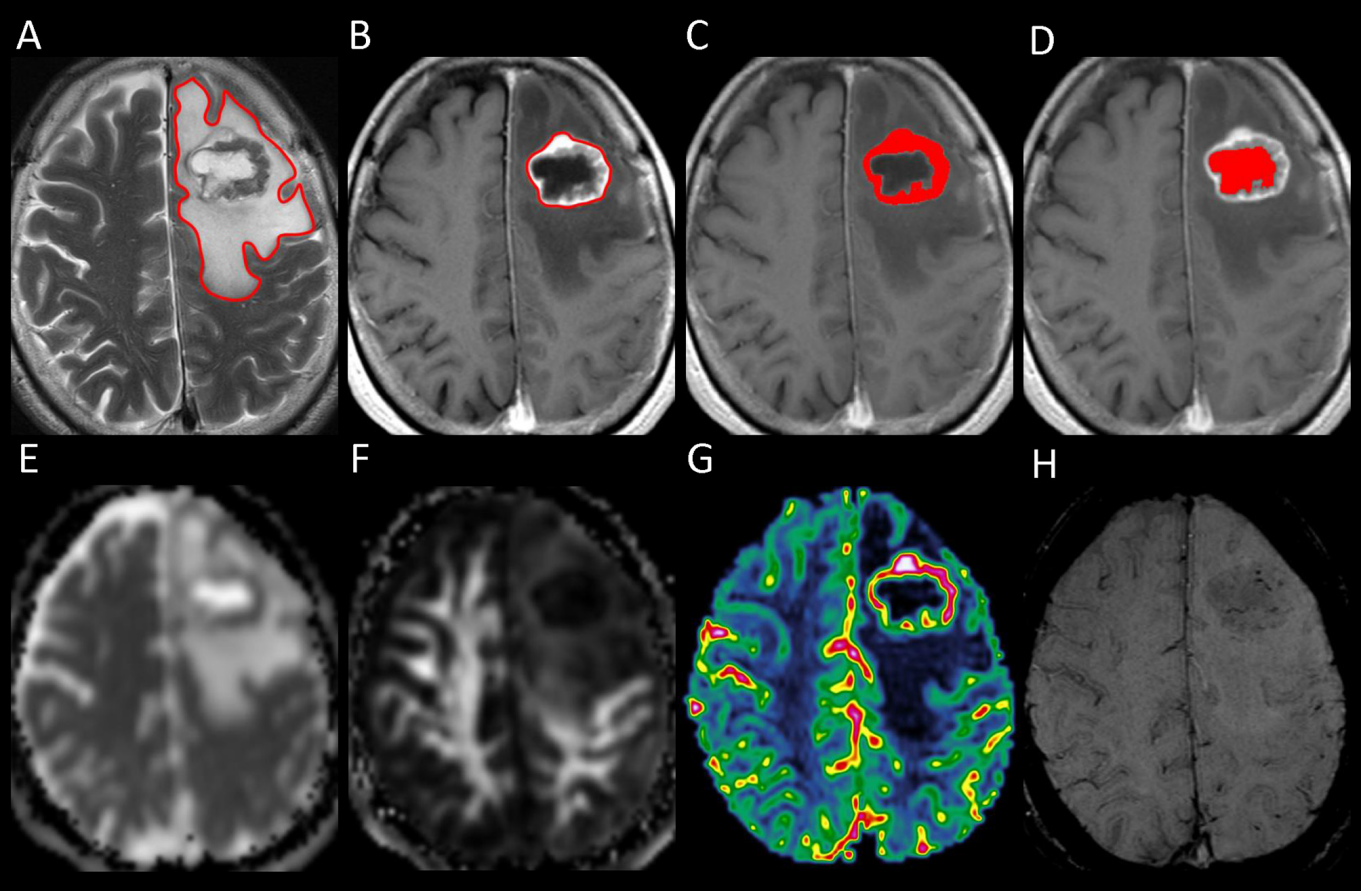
Example of how regions of interest (ROIs) were segmented in a left frontal metastatic brain tumor. Transverse T2-weighted image (A) shows a manually drawn polygonal ROI that includes the entire peritumoral brain edema and the whole tumor. On contrast-enhanced T1-weighted images (B–D), the ROIs of whole tumor (B), enhancing tumor (C), and necrotic portion (D) are shown. The ROI of the enhancing tumor (C) is used to measure the tumor apparent diffusion coefficient (ADC), tumor fractional anisotropy (FA), and tumor cerebral blood volume (CBV) by overlaying the ROI on the corresponding ADC (E), FA (F), and CBV (G) maps. On susceptibility-weighted image (H), there is no hemorrhagic tumor portion.
